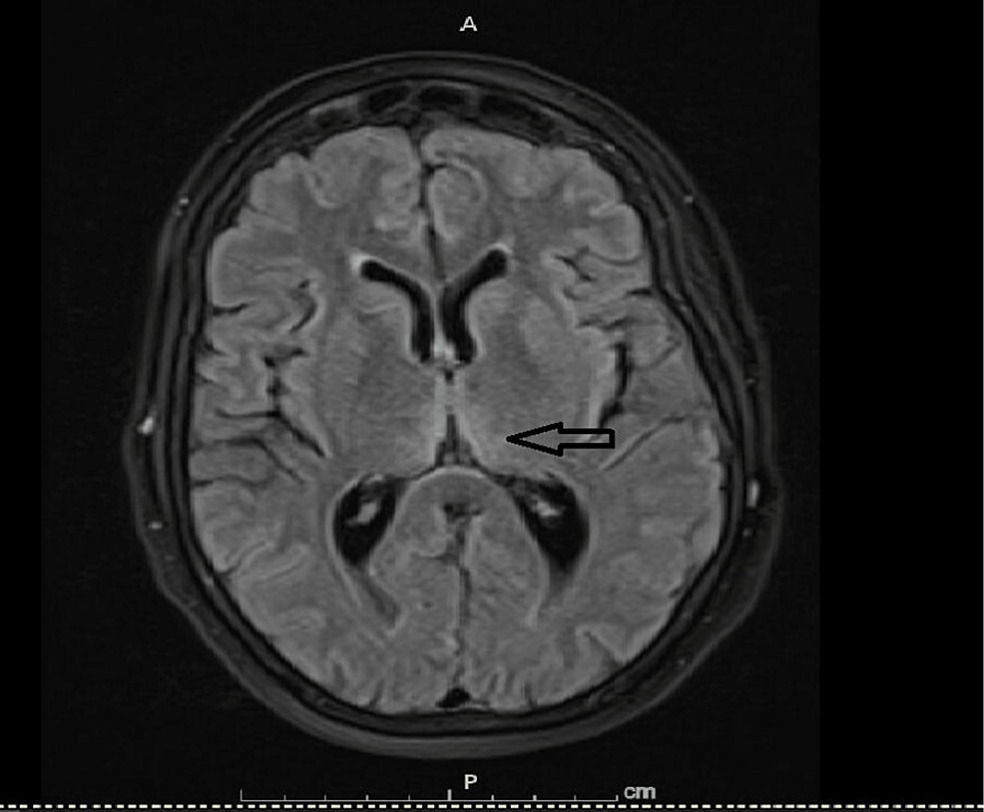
FLAIR MRI medial thalamic hyperintensities FLAIR MRI of the brain shows vague bilateral hyperintensities in both medial thalami (arrow).
